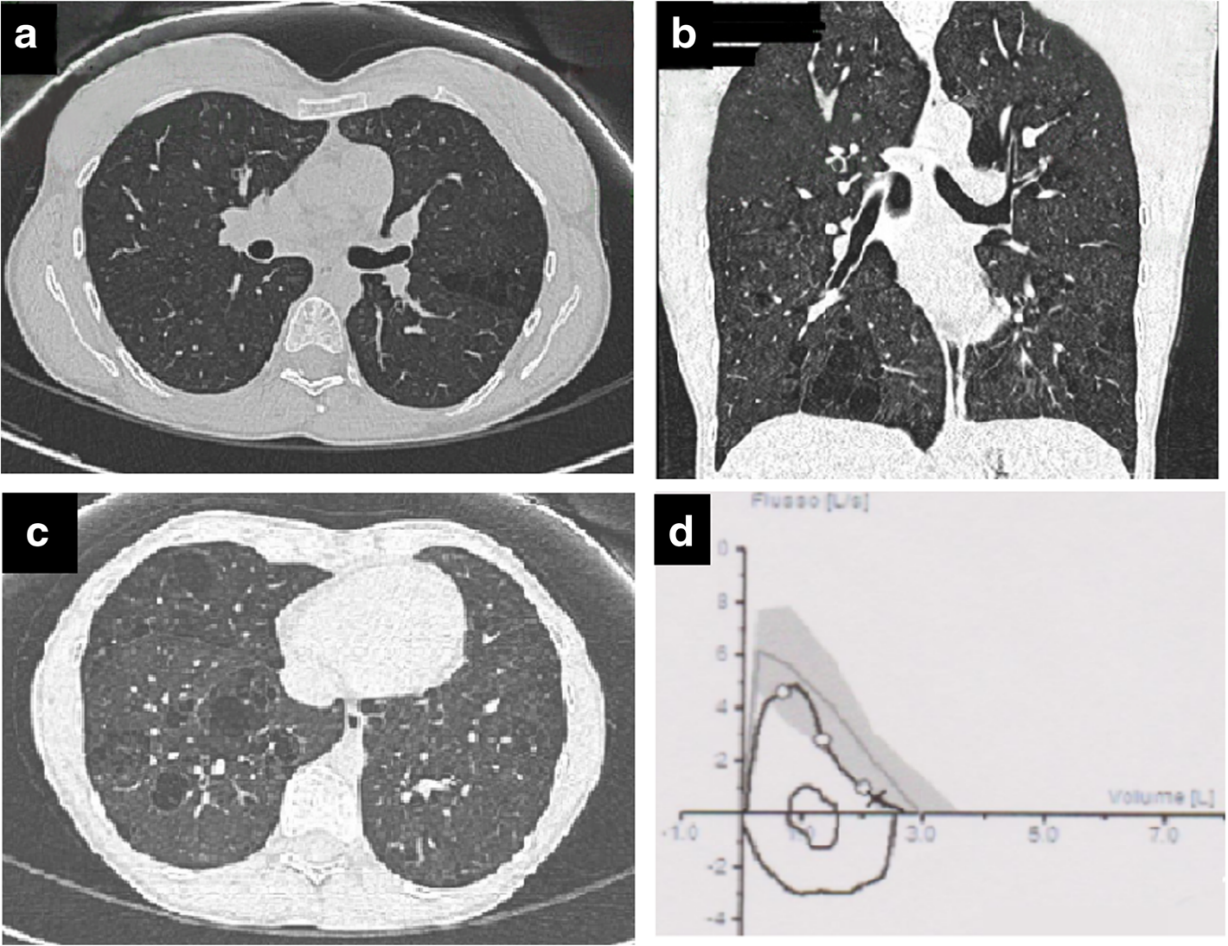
Panel a, b, c: HRCT scan images demonstrating atypical paraseptal emphysema; Panel d: flow-volume loops showing normal values
